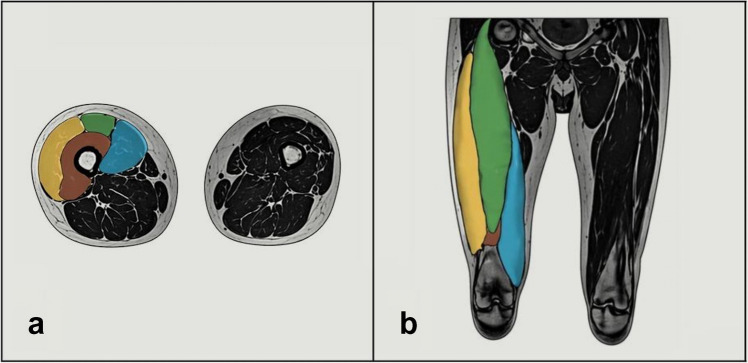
Volumetric measurement of quadriceps muscles on magnetic resonance imaging (MRI). Axial MRI slice demonstrating manual segmentation of the individual quadriceps muscles: rectus femoris, vastus lateralis, vastus intermedius, and vastus medialis a. Three-dimensional reconstruction of the segmented quadriceps muscles, enabling volumetric measurements b
